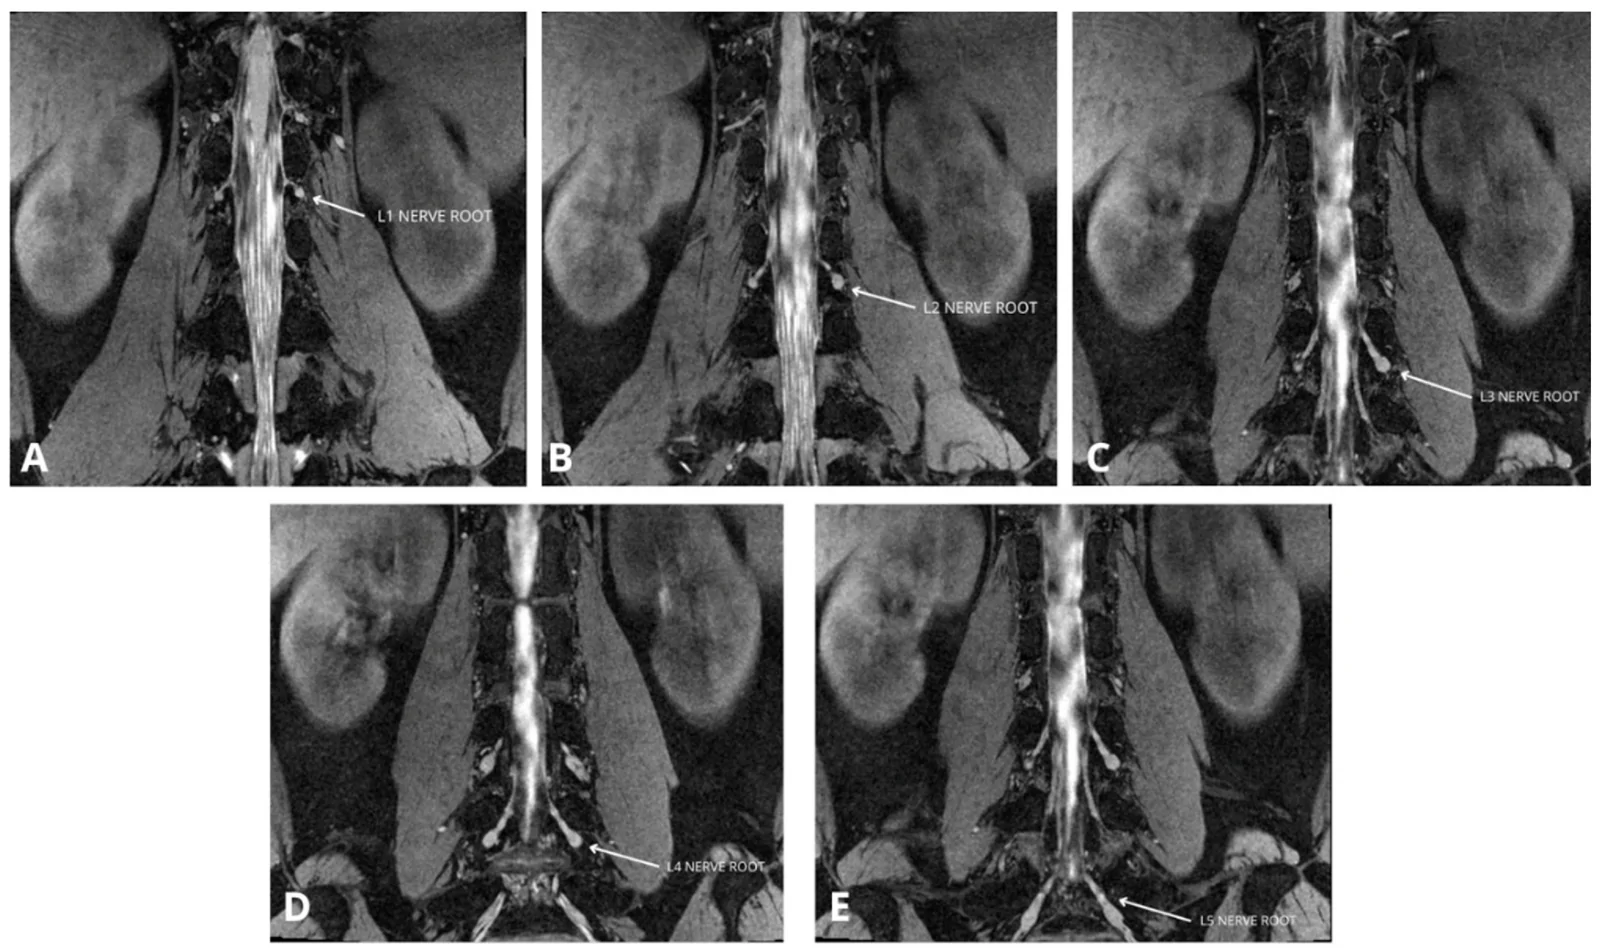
Coronal fat-suppressed T2-weighted MR neurography of the lumbar plexus in a healthy subject, demonstrating sequential identification of the individual nerve roots contributing to the plexus: L1 (A), L2 (B), L3 (C), L4 (D), and L5 (E). Original Image.
